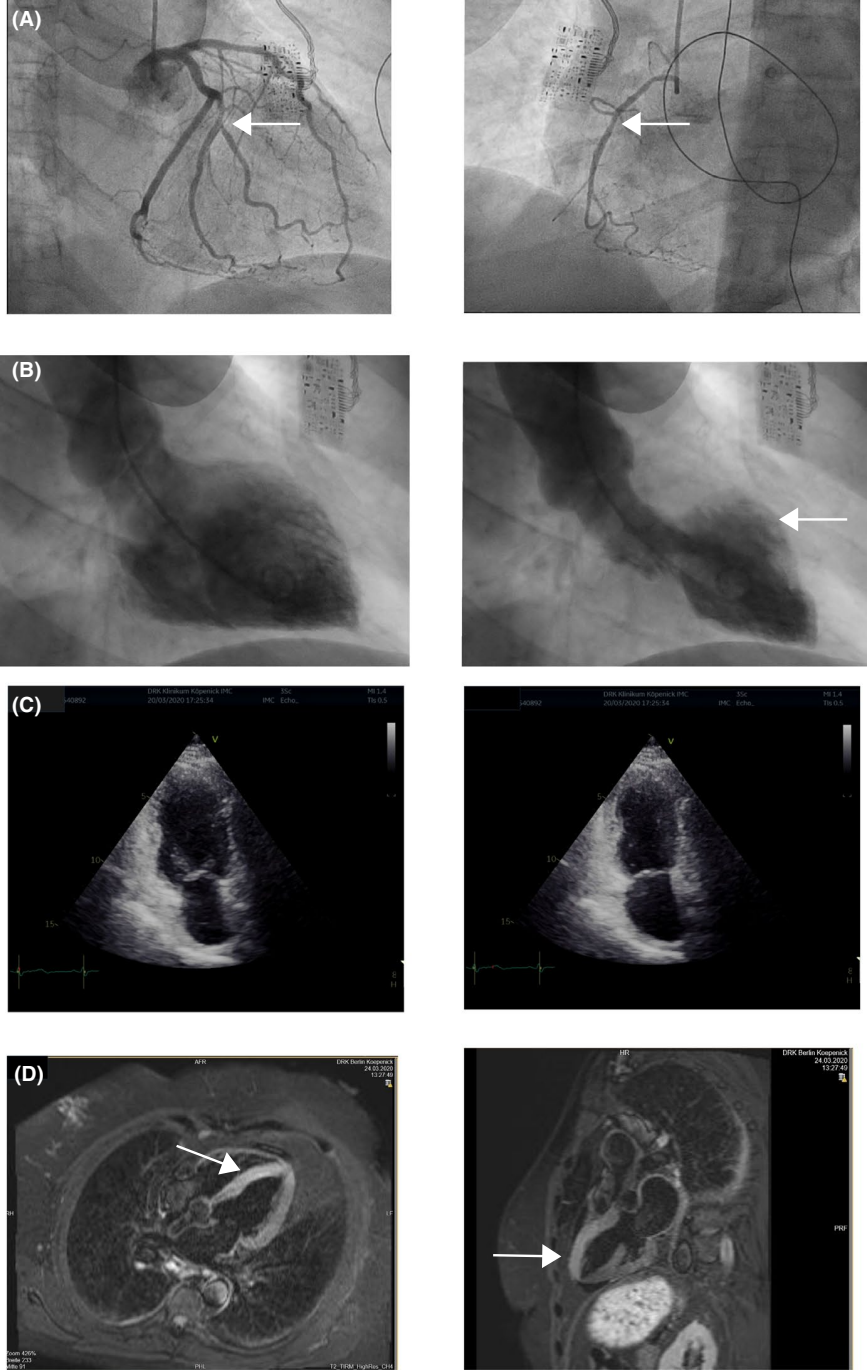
Images. A, Coronary angiogram (arrows: sclerosis in the right coronary and left circumflex artery). B, Ventricular angiogram (arrow: mid‐anterior pronounced akinesia). C, Echocardiography 24 h after VF (left: diastole, right: systole). D, Cardiac MRI (arrow: edema of the anterior wall)
